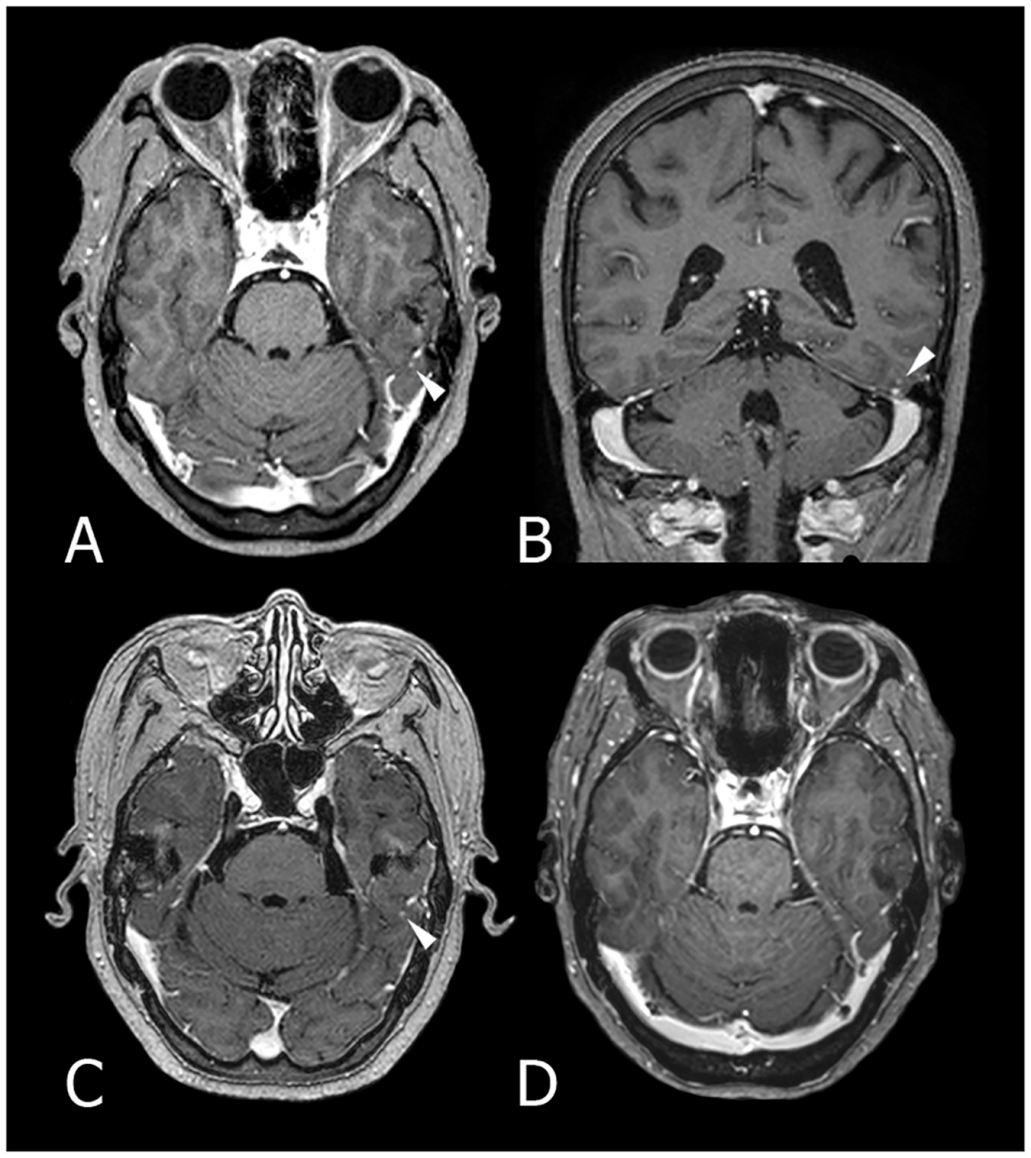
3D gradient-echo contrast-enhanced T1-weighted MR images in an 81-year-old female patient with metastatic lung cancer. A and B: Axial (A) and coronal (B) images show a tiny enhancing nodule at the left inferior temporal gyrus (arrowhead). This nodule was missed by all four reviewers but was successfully detected by CAD. C: On the navigation MR image for a gamma-knife surgery performed 2 days after (A) and (B), the nodule showed no interval changes. D: On the follow-up MR image taken after 3 months, the nodule disappeared.
